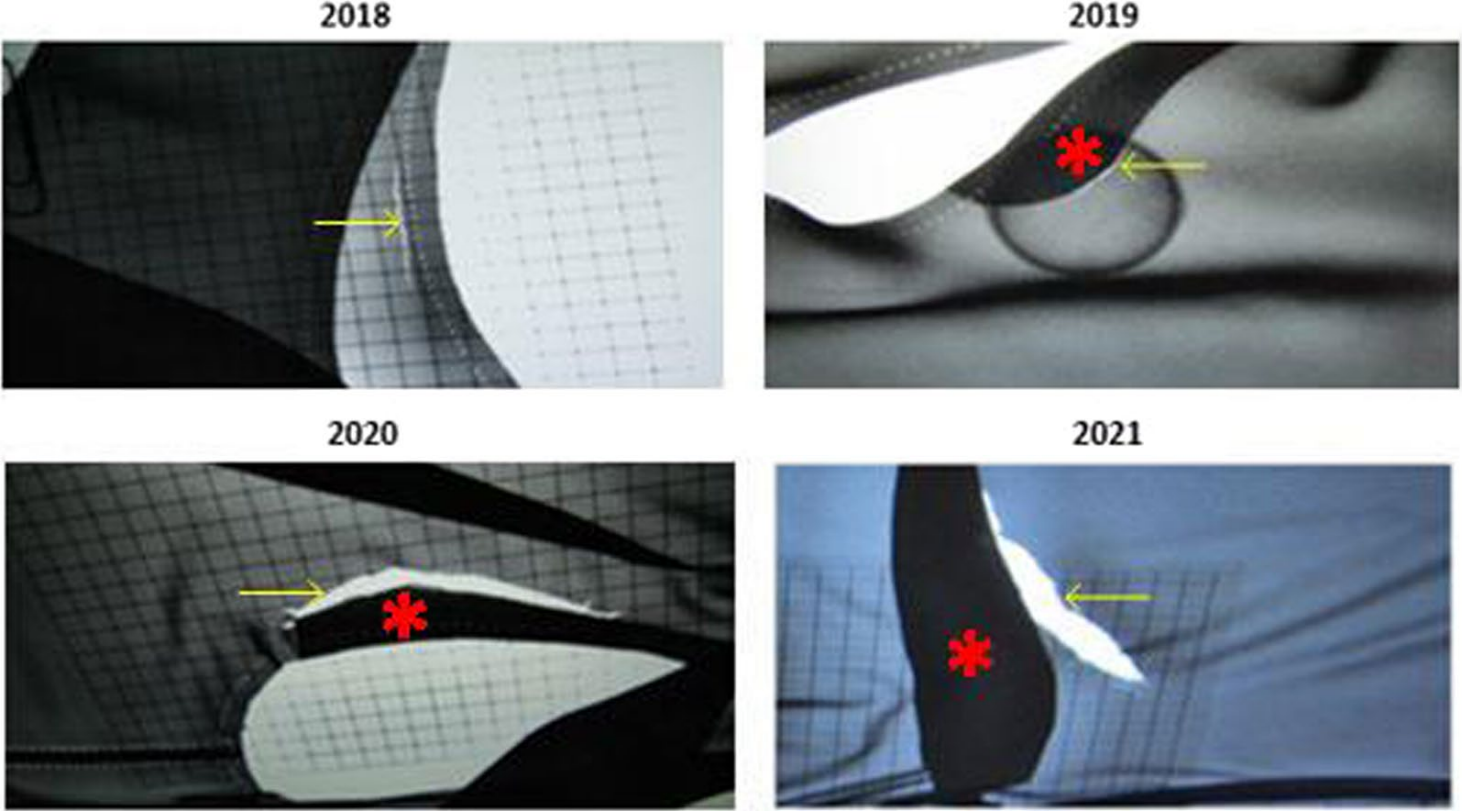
Tear (yellow arrow) evolution in a lead-free vest that was sent yearly to the manufacturer for repair. The X-ray opaque grid to measure the tears is seen in the upper left, bottom left and bottom right picture. The dark patch (red asterisk) is a piece of lead-free fabric that is placed over the tear at the left arm hole. In 2019, a new tear emerged right beside the repair patch. The round sphere shows the tennis ball to identify the tear more easily. In 2020, after two repairs, a big tear of ± 300 mm^2^ is clearly visible. In 2021, after three repairs, another big tear formed beside the repair patch
